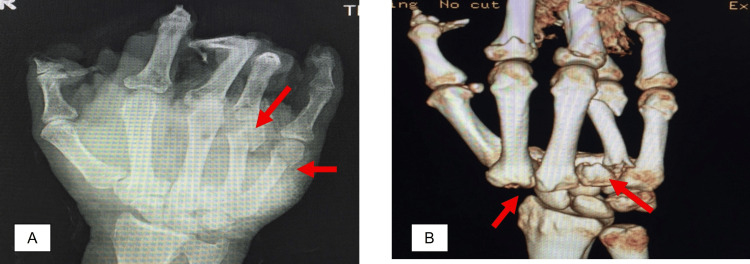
Preoperative radiographs and CT scan Anteroposterior radiographs (A) and a coronal CT scan (B) demonstrating multiple fractures of the metacarpals and phalanges, as well as multiple CMC fracture-dislocations (red arrows). CMC, carpometacarpal
